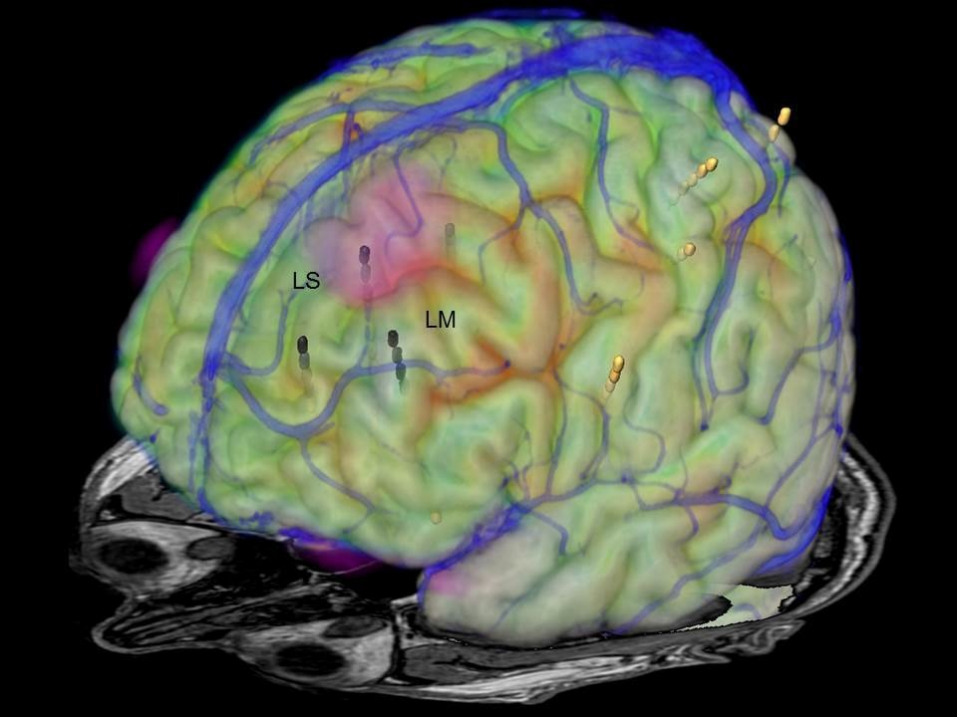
Image fusion of superficial veins, MRI, FDG-PET, DTI-MRI and depth electrodes Superimposition of the superficial veins (blue) on MRI, FDG-PET, DTI-MRI, and the depth electrodes (yellow electrodes). The seizure onset zone and regions of maximal interictal epileptiform discharges are in the electrodes marked black. LS: left superior frontal gyrus; LM: left middle frontal gyrus; MRI: magnetic resonance imaging; FDG-PET: fluorodeoxyglucose positron emission tomography; DTI: diffusion tensor imaging
